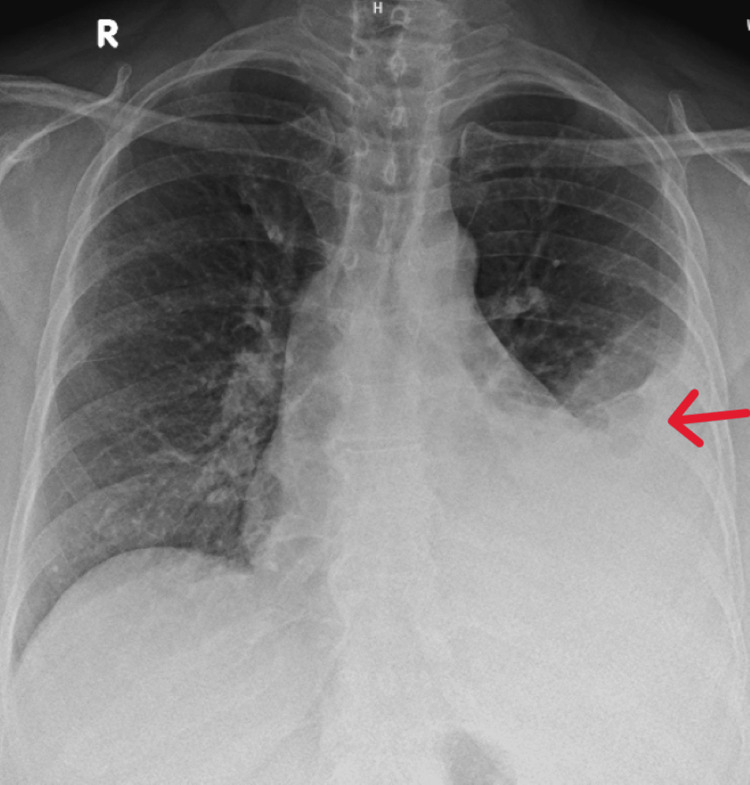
Initial chest radiograph demonstrating homogeneous opacification of the left mid and lower lung zones with blunting of the left costophrenic angle (arrow), consistent with left-sided pleural effusion.
